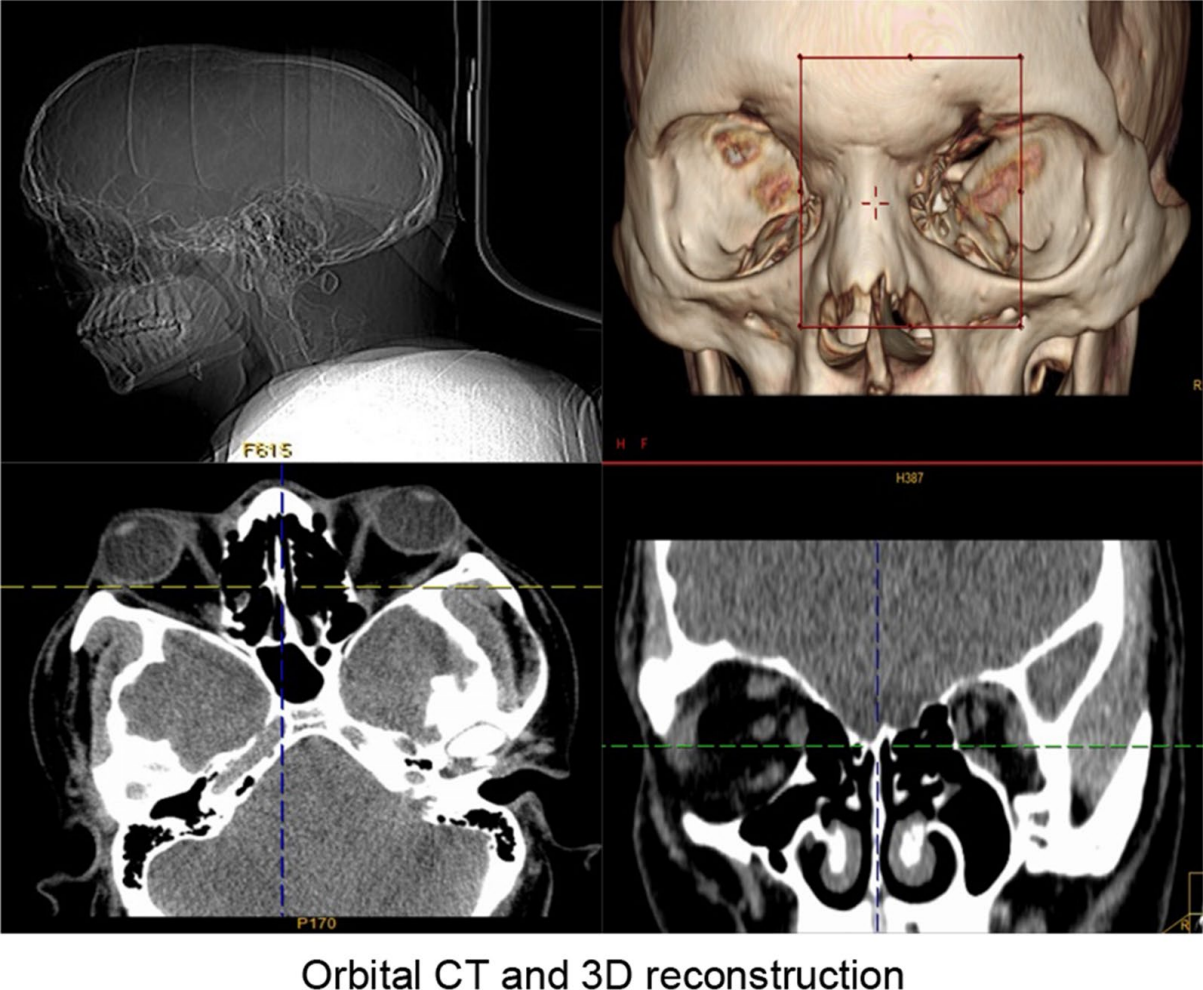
CT scans of the orbits and a three-dimensional reconstruction of the orbits showing shallowed orbits, prominent eyeballs, and slight medial shifts in recti muscles within both eyes
